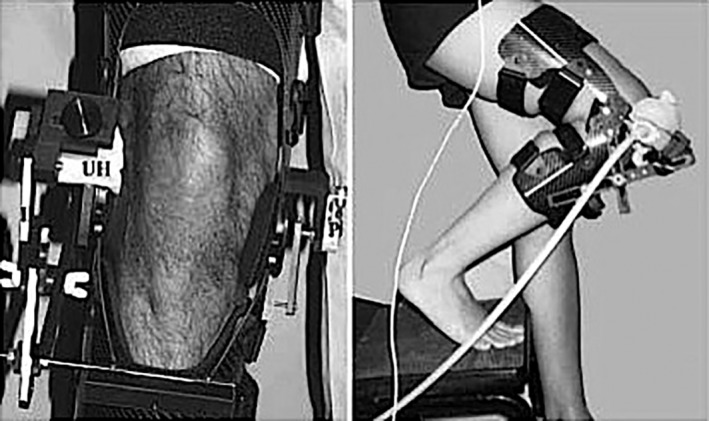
Shih custom‐made device attaching an ultrasound probe to a knee brace^27^.
